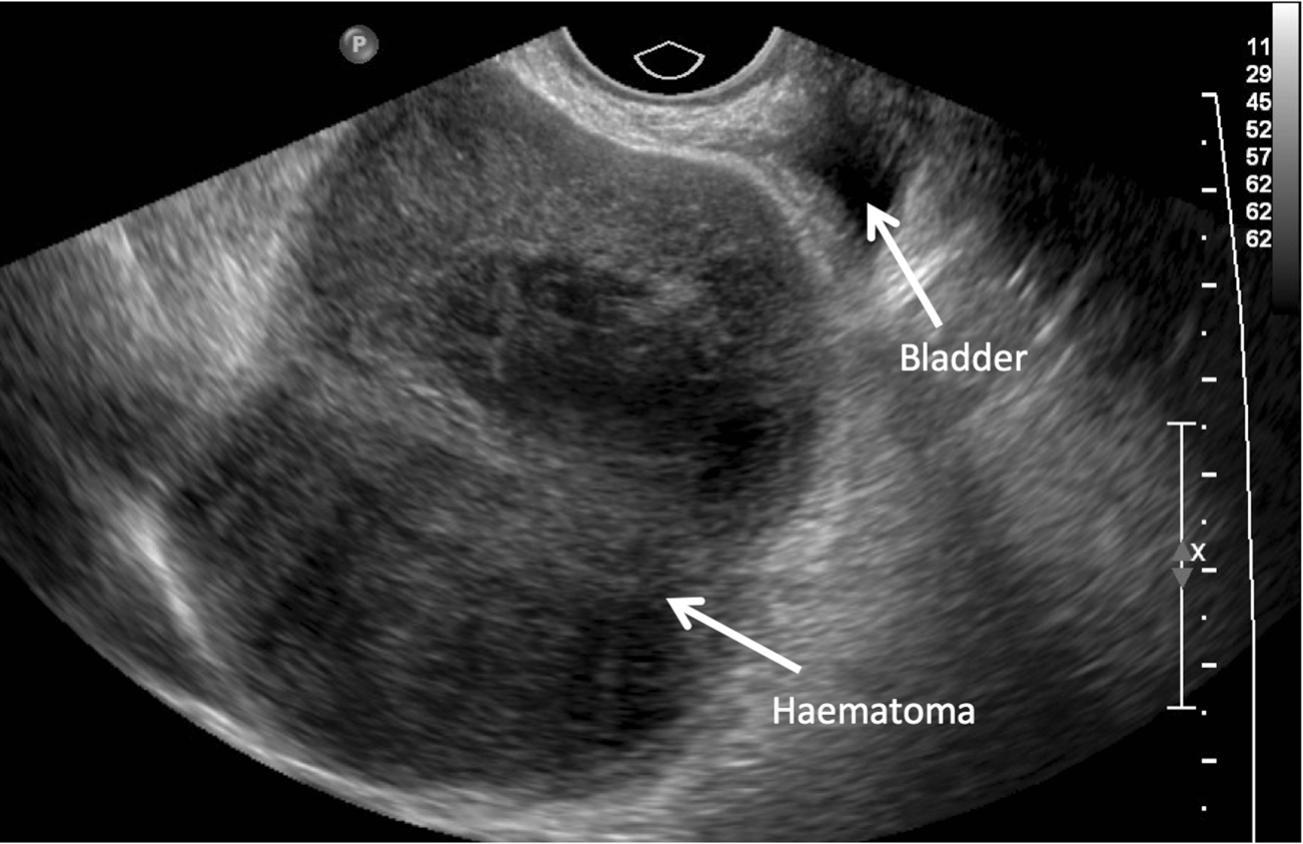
Predrainage transvaginal ultrasound scan of haematoma = 9 cm (ultrasound depth 8.1 cm)
